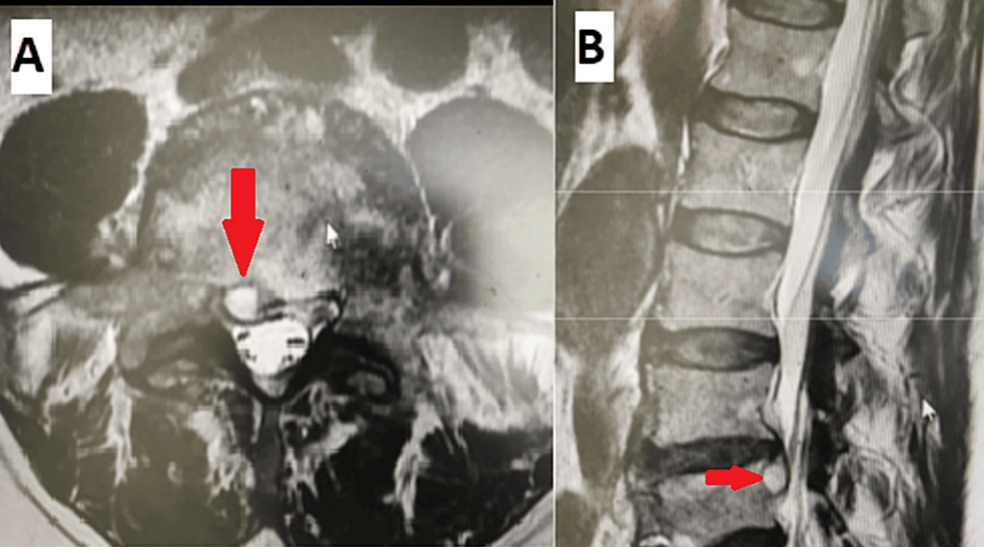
(A) Axial and (B) sagittal MRI of a 55-year-old male patient with right radicular symptoms secondary to discal cyst (red arrow).
